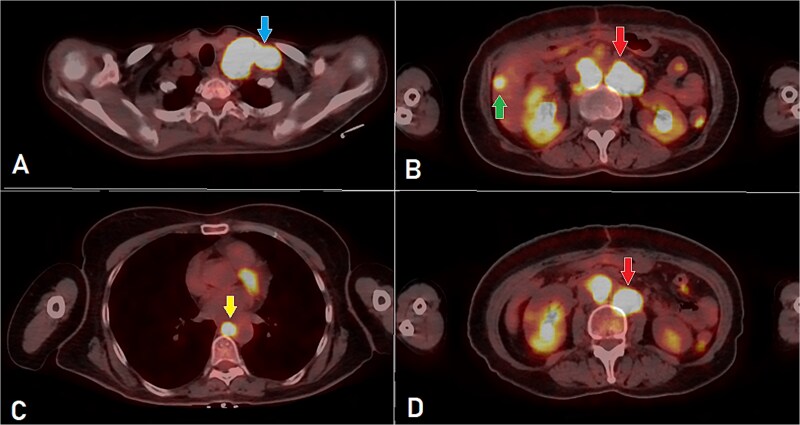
PET-CT scan (A) showing matted intensely hyper-metabolic left supraclavicular lymph nodes (arrow); (B) demonstrating intensely hyper-metabolic retroperitoneal lymph nodes (inferiorly pointing arrow) and a hyper-metabolic solitary hepatic lesion (superiorly pointing arrow); (C) demonstrating an intensely hyper metabolic mediastinal (para- oesophageal – station VIII) lymph node (arrow); and (D) revealing a few intensely hyper-metabolic retroperitoneal lymph nodes (arrow).
